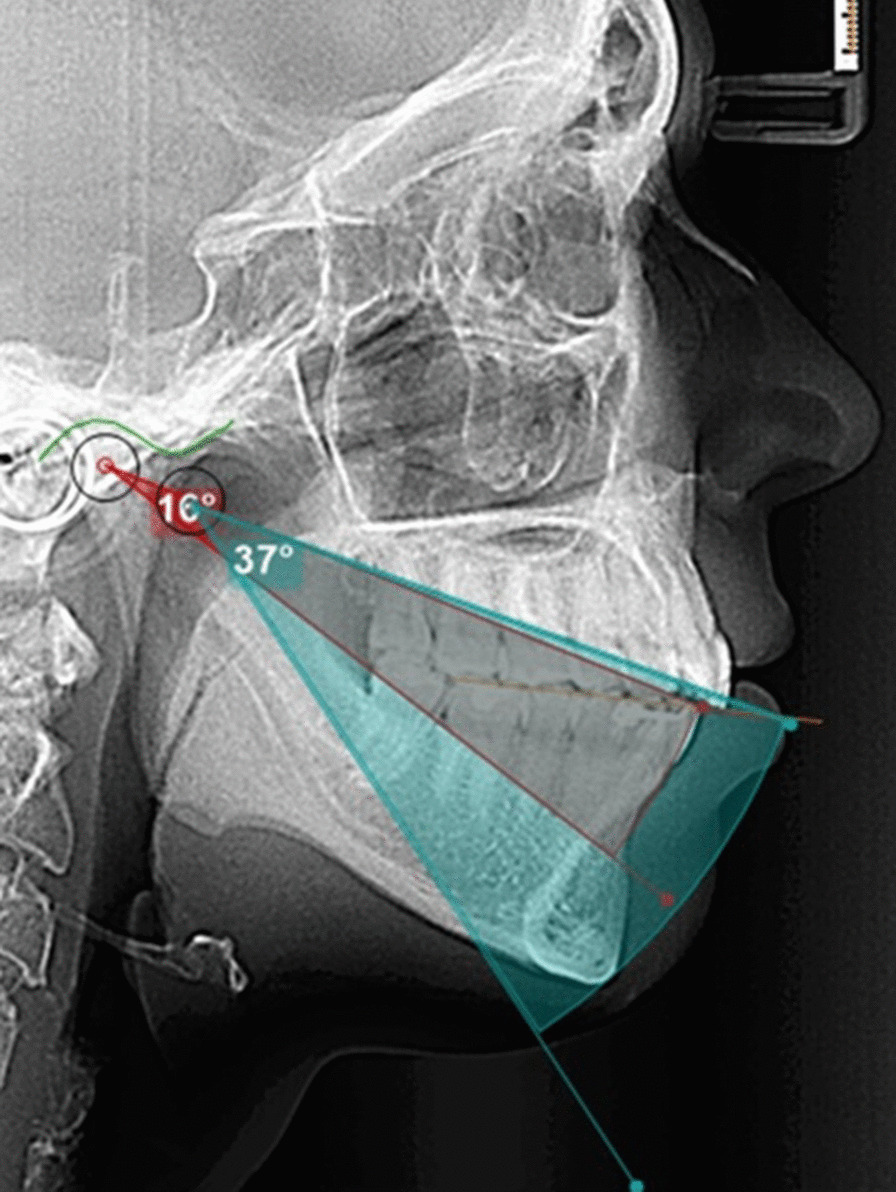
Condyle position at maximum retrusion and advancement. The condyle position at maximum advancement (blue arch) and retrusion (red arch) calculated considering the initial position of it in the radiograph and the morphology of the glenoid fossa
